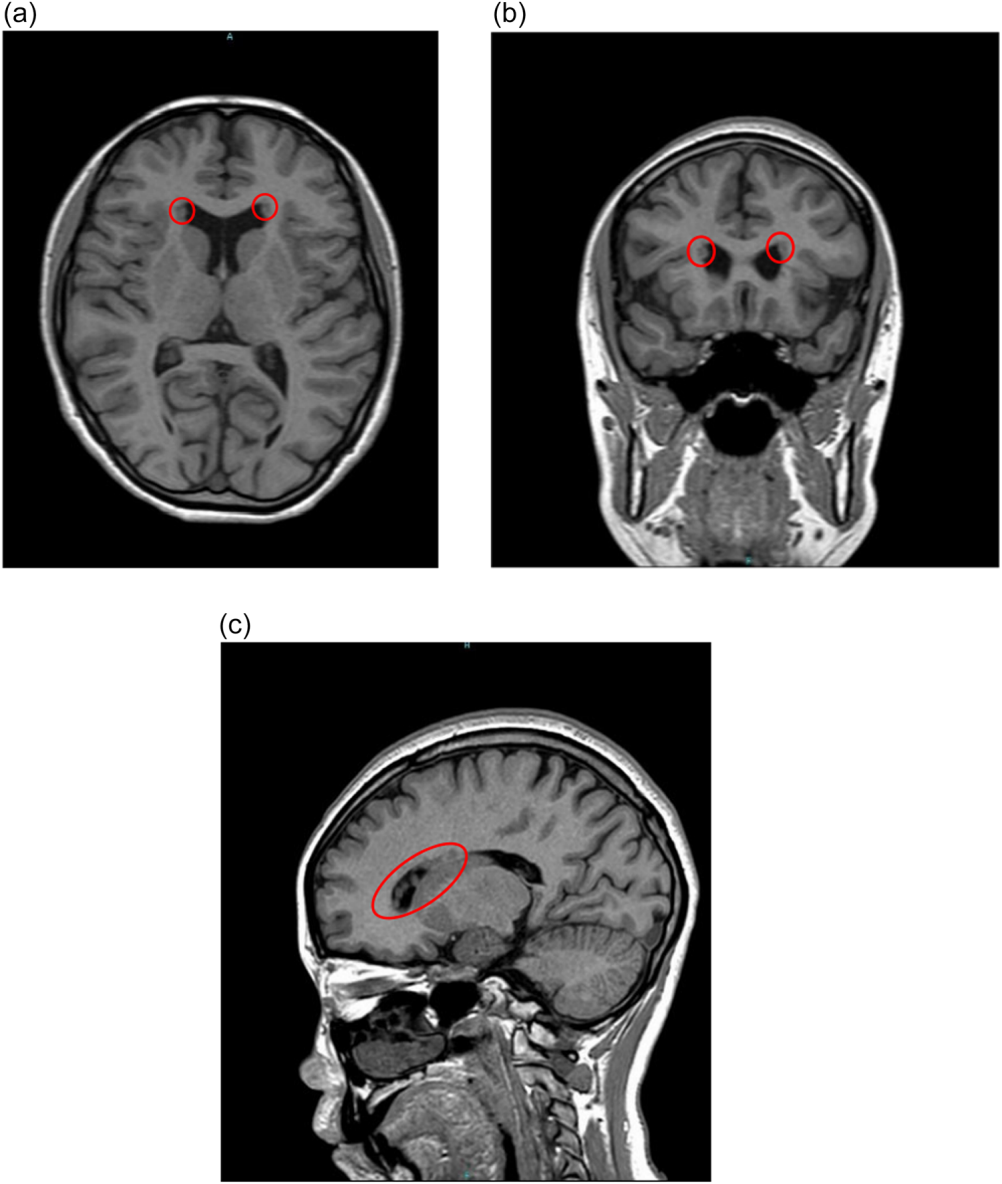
(a–c) Magnetic resonance imaging (MRI) findings. Brain MRI revealed bilateral, multiple periventricular nodular heterotopia (PNH) located at the dorsal pole of the frontal horn of the lateral ventricles. (a) Axial T1‐weighted image, (b) coronal view, and (c) sagittal view. The sagittal image (c) shows multiple PNHs along the lateral ventricular walls. The area circled in red indicates the locations of the PNHs.
